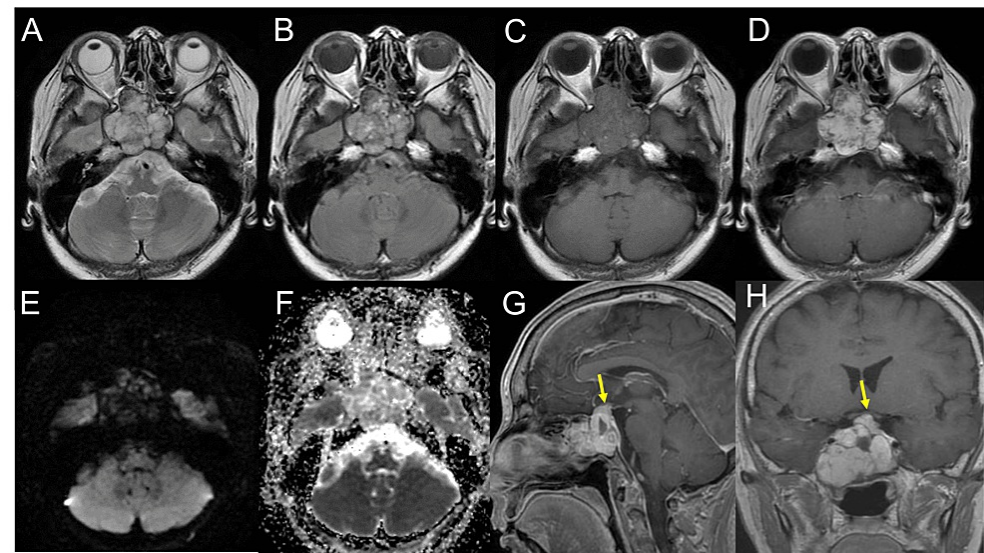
Preoperative magnetic resonance imaging On the magnetic resonance imaging (MRI) scan, the lesion shows predominantly hyperintensity with hypointense, thin internal septal structures on the T2-weighted image (A) and fluid-attenuated inversion recovery (B). The pre- (C) and post-contrast (D) T1-weighted image (T1WI) revealed the avid enhancement of the mass. The lesion did not show restrictions on the diffusion-weighted image (E) or apparent diffusion coefficient map (F). On sagittal (G) and coronal (H) post-contrast T1WI, the lesion was located mainly at the sphenoid sinus, involving the cavernous sinus and the sella. The optic chiasm was compressed by the lesion (G, H, yellow arrow).
